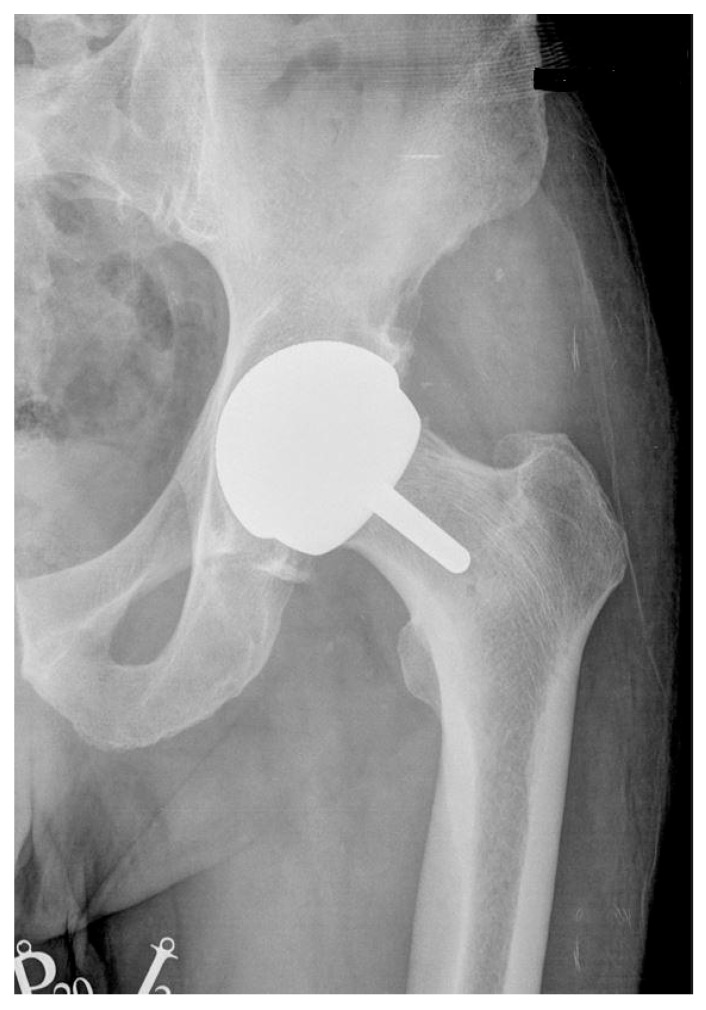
X-ray scan showing the hip operated with use of BHR (Smith & Nephew, Watford, UK).
